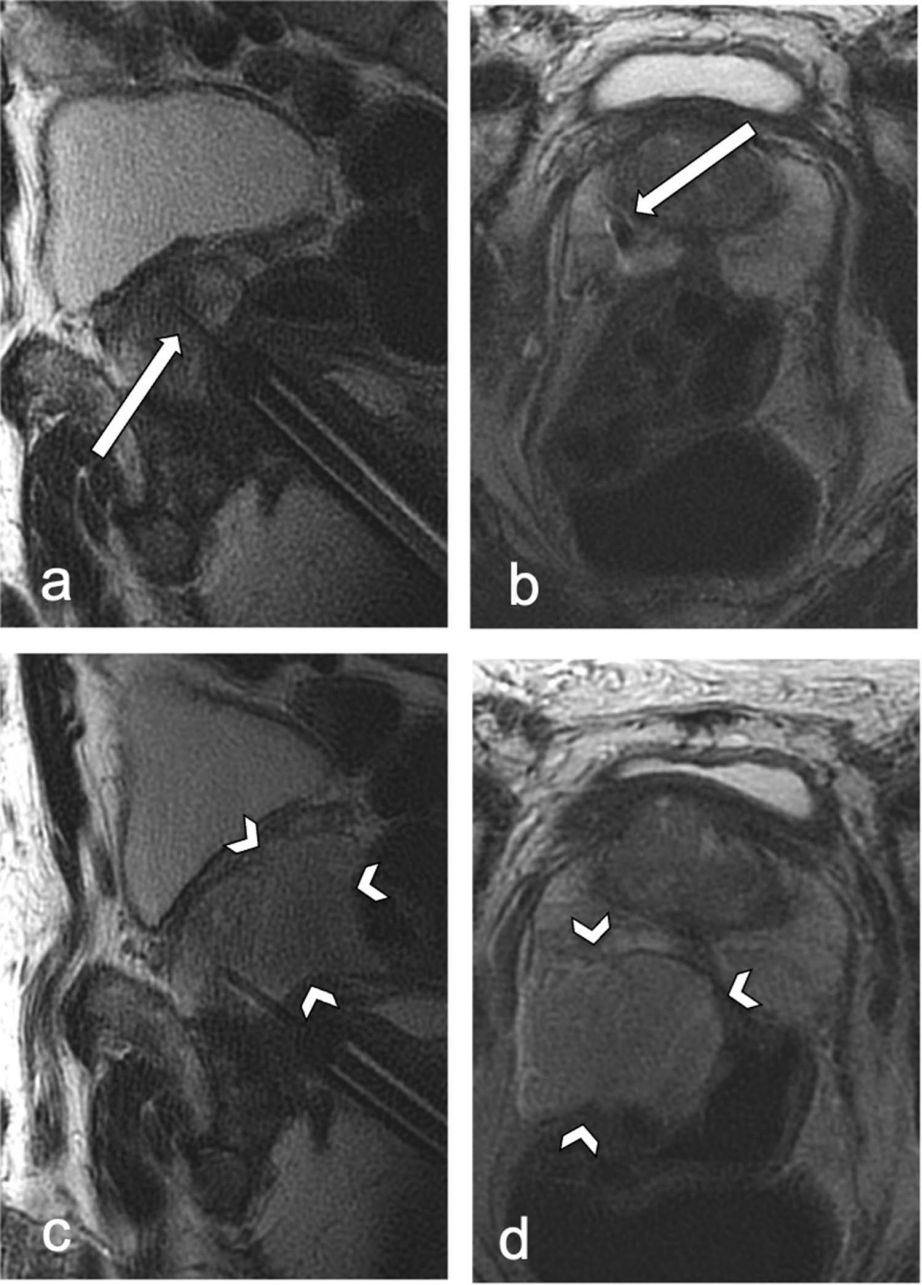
Large rectoprostatic hematoma of 40 ml (arrowheads); sagittal (a,c) and axial (b,d) T2-weighted fast imaging in a 63 years old patient without anticoagulants nor antiplatelet therapy; (a,b) first intraprostatic needle documentation, inside the peripheral zone on the right (arrows); (c,d) needle documentation at the end of the intervention.
